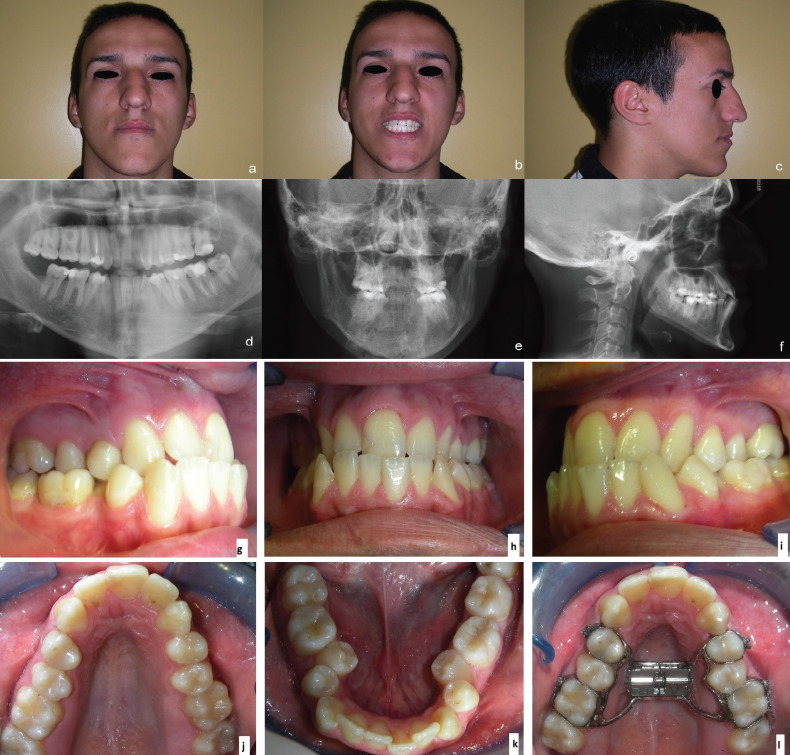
Initial pictures of the patient (a-c). Panoramic radiograph (d). Posterior-anterior radiograph (e). Latero-lateral radiograph (f). Right lateral view (g), frontal view (h), left lateral view (i), palatal view (j), lingual view (k), expander appliance cemented on first premolars and molars (l).
